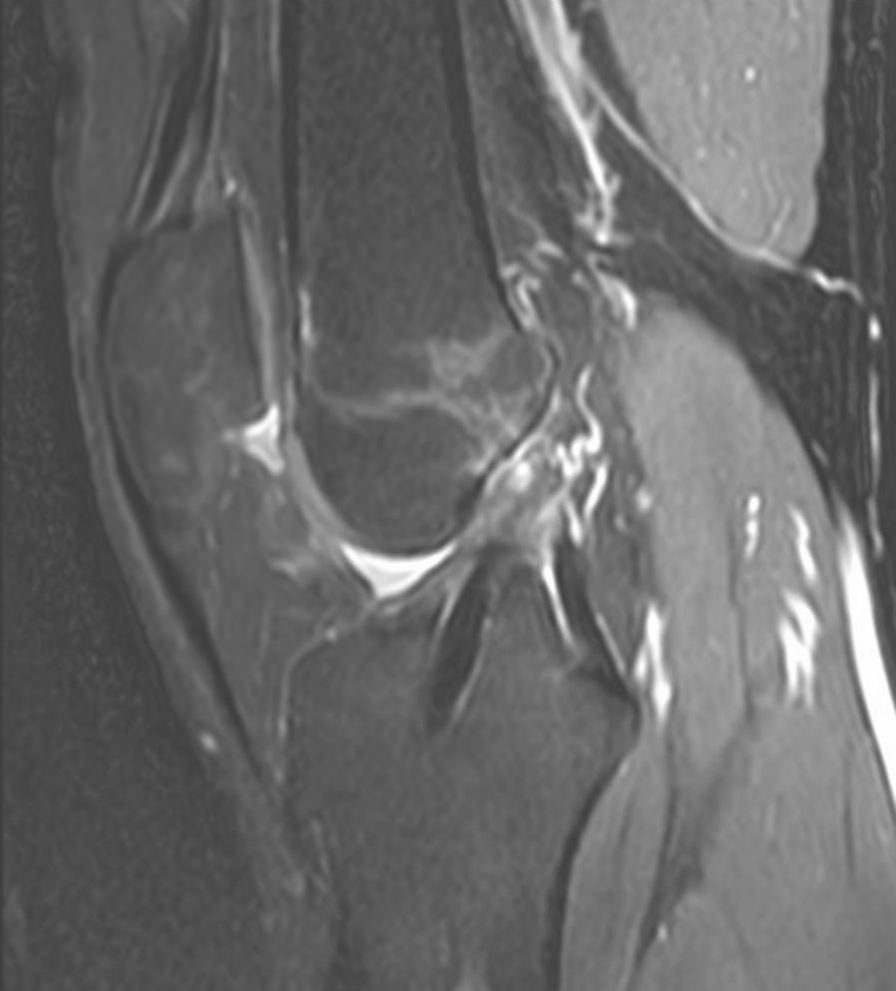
Sagittal magnetic resonance imaging of the fibrous interzone in the tibial tunnel in STGPI graft
